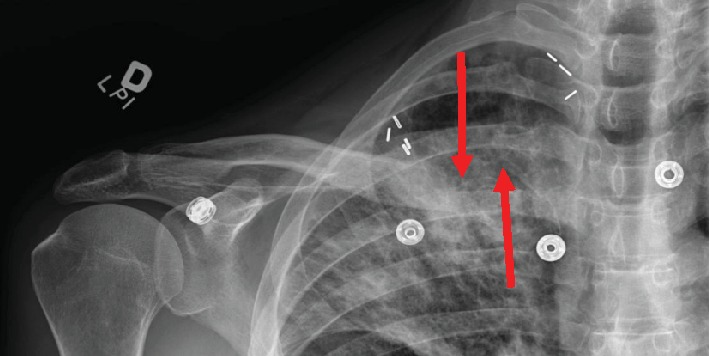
Clavicle radiograph five months after the initial injury showing a right medial clavicle fracture nonunion (red arrows). Vascular clips are from a rib resection at a young age.
